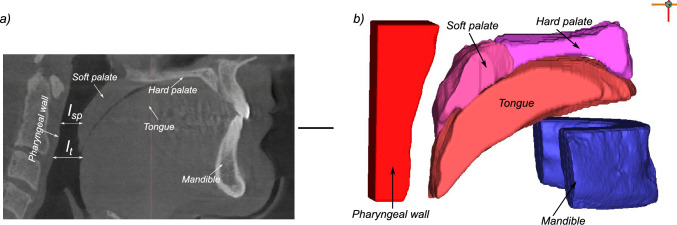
a Midsagittal section of the CT image scan of patient 1; b end result of the segmentation process in MIMICS, with the different tissues of interest marked separately
